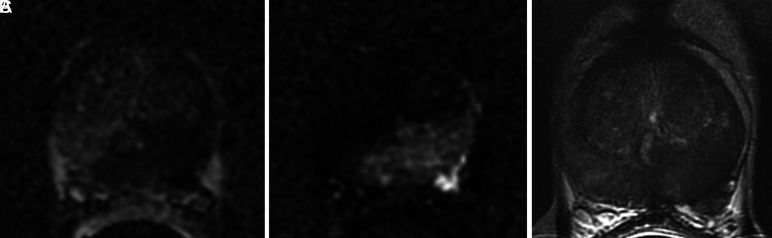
Magnetic resonance images of axial sequences ADC (A), DWI (B), and T2WI (C) of obliteration of the recto-prostatic angle. There is evidence of a large lesion that infiltrates the periprostatic fat in the peripheral area, more evident on the left side with a solid token in the left postero-lateral region. The lesion shows marked hyperintensity in DWI and marked hypointensity in the ADC map. ADC, apparent diffusion coefficient; DWI, diffusion-weighted imaging.
